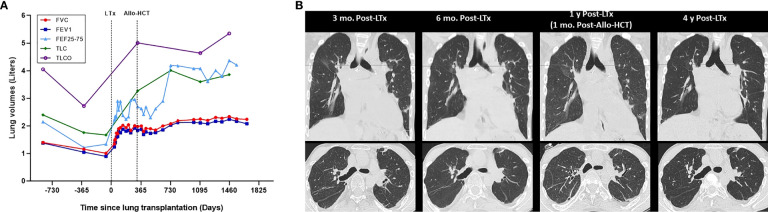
Evolution of pulmonary function and chest CT over time. (A) Pulmonary function. (B) Chest CT. Evolution of pulmonary function over time in relation to lung transplantation (LTx) and allogeneic hematopoietic stem cell (Allo-HCT) for hereditary pulmonary alveolar proteinosis (A), which are indicated by dotted lines. Dots represent individual measurements of lung volumes (Liters), with connecting lines between measurements showing the trend of individual lung function parameters over time. Evolution of chest computed tomography (CT) function over time (B), with representative inspiratory phase images at 3 months, 6 months 1 year and 4 years post-lung transplantation (LTx). Allogeneic hematopoietic stem cell (Allo-HCT) was performed 11 months after prior lung transplantation. At 4.5 years after lung transplantation there is currently no evidence for chronic lung allograft dysfunction, graft-versus-host disease, or recurrence of hereditary pulmonary alveolar proteinosis. FVC, forced vital capacity; FEV1, forced expiratory volume in one second; FEF25-75, forced expiratory flow at 25–75% of forced vital capacity; TLC, total lung capacity; TLCO, transfer capacity of the lung, for the uptake of carbon monoxide (CO) (also called diffusing capacity for carbon monoxide).
